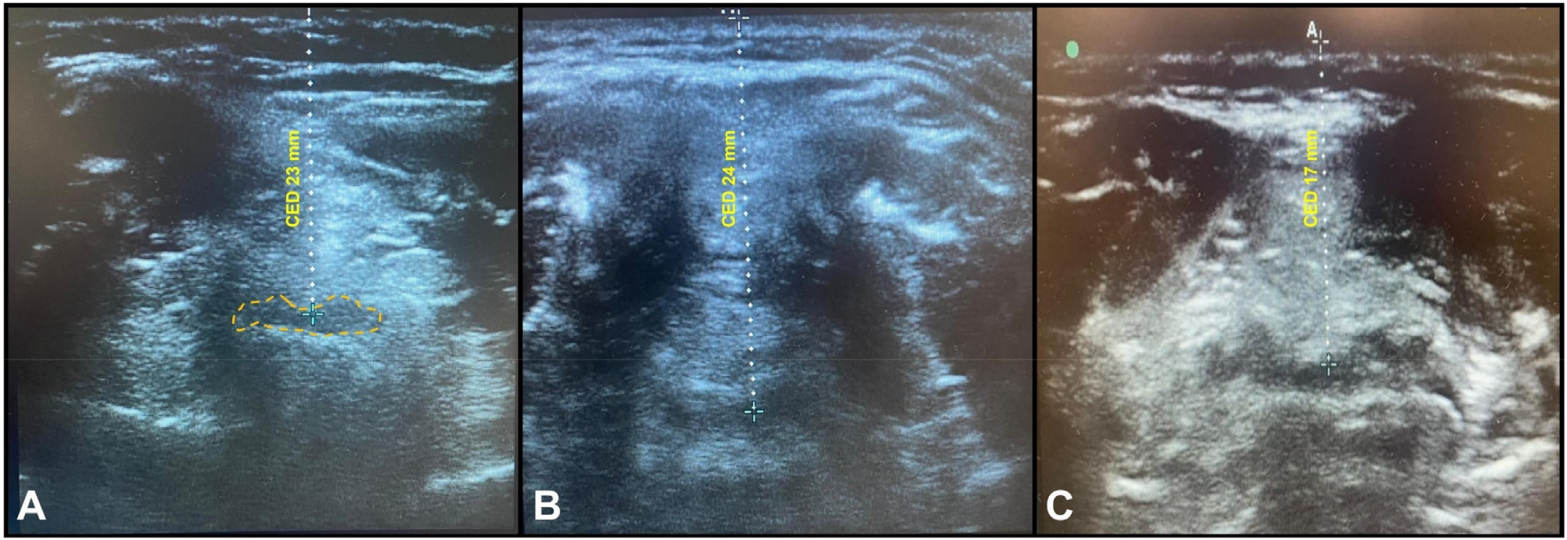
Airway ultrasound images and respective cutaneous-epiglottic distance (CED) measurements. The epiglottis is outlined (dashed orange line) in panel A.
